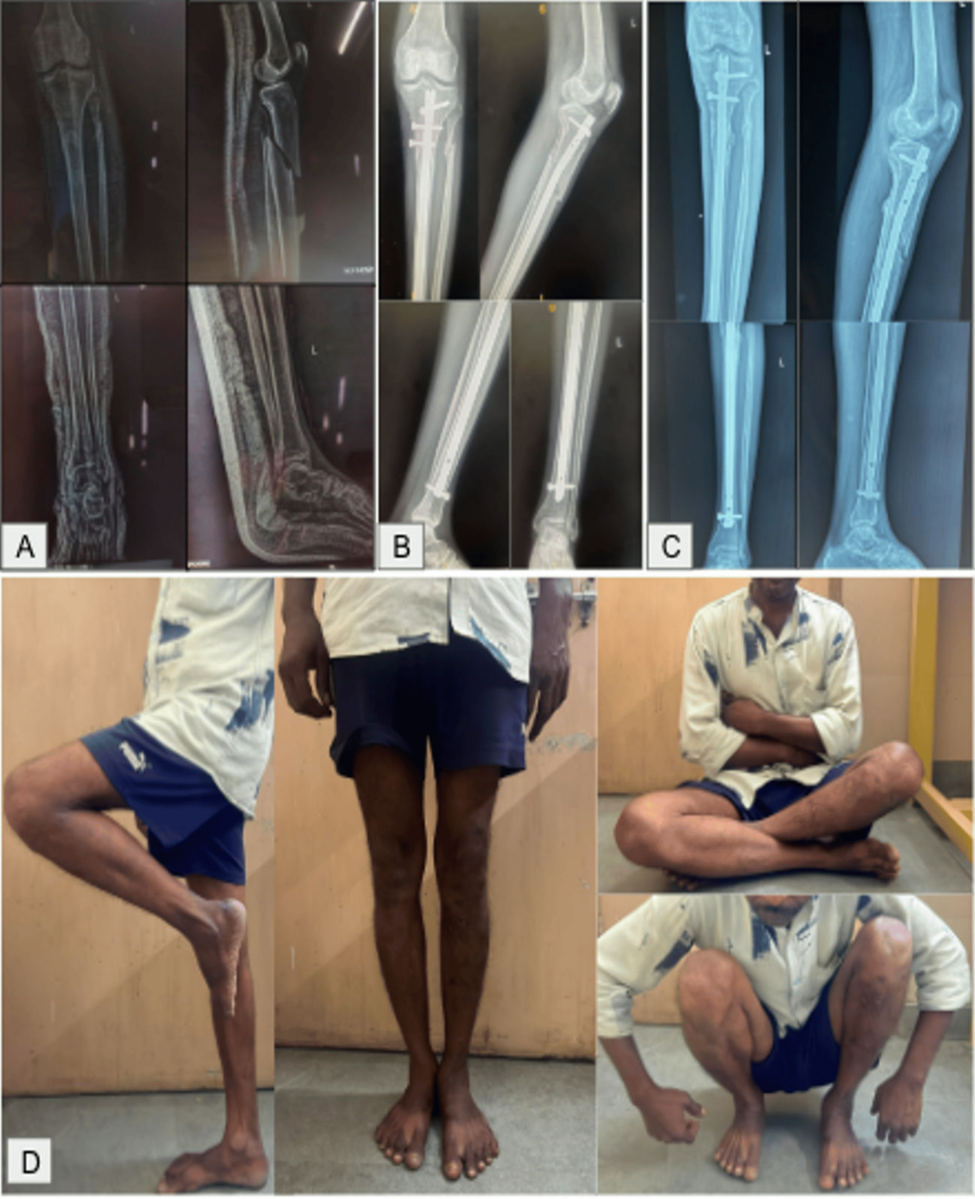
Case 1 of suprapatellar nailing. (A) Preoperative X-ray. (B) Immediate postoperative X-rays. (C) Three months postoperative X-rays. (D) Clinical assessment and knee range of motion at three months.
